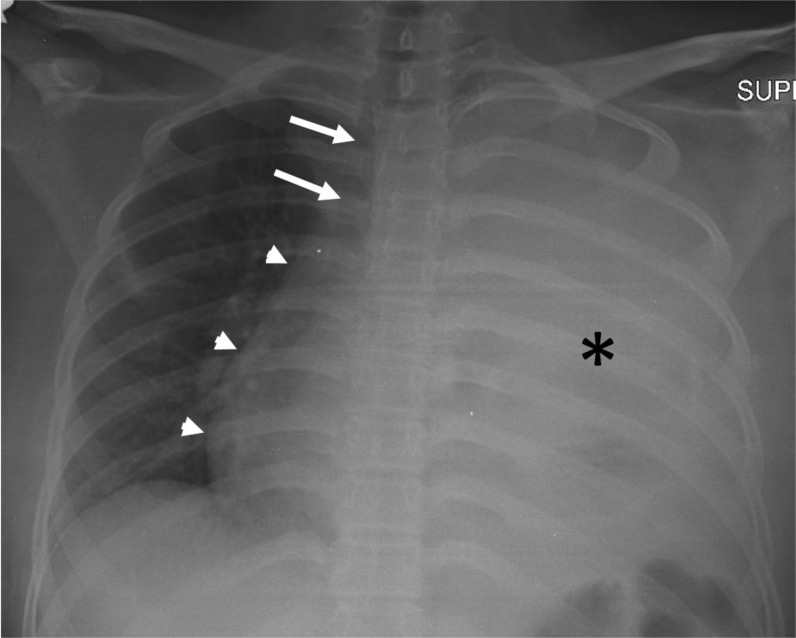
Chest radiography demonstrates opacity occupying the left hemithorax (*) causing obliteration of the left heart border, aortic knuckle, and left hemidiaphgram. The mediastinum (arrowheads) and trachea (arrows) shifted to the right.
